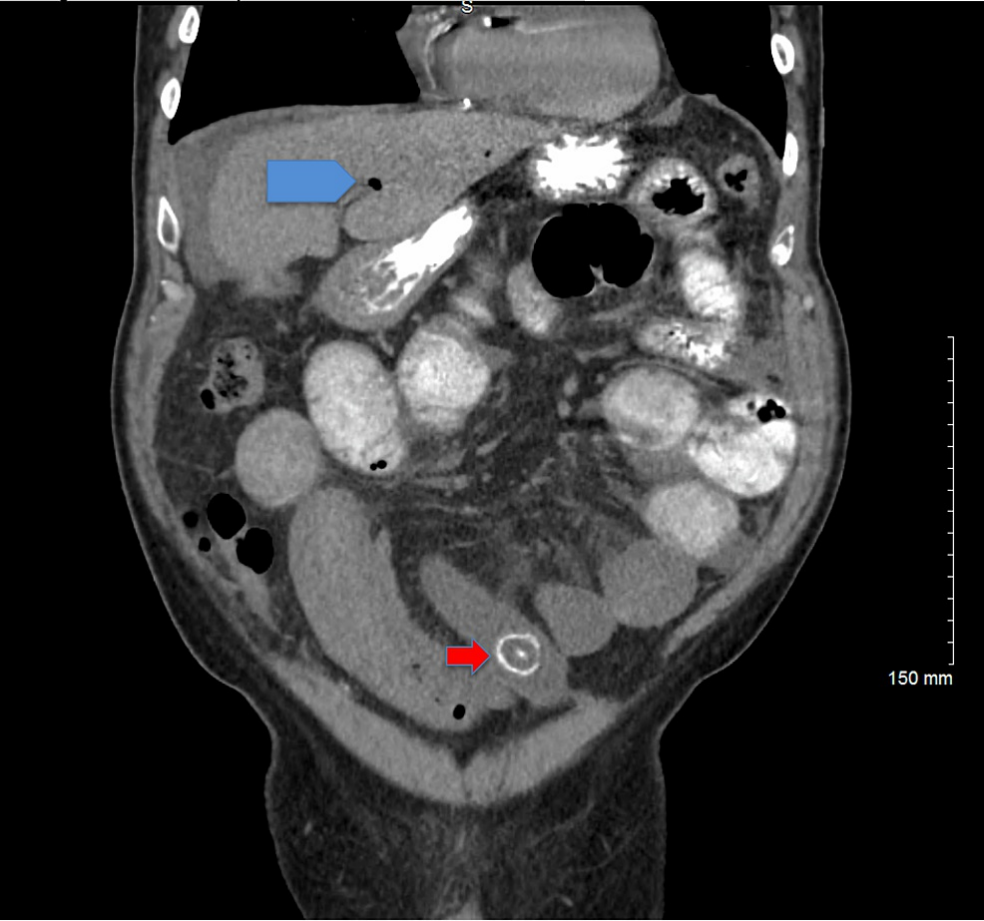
Coronal CT of abdomen and pelvis showing dilated small bowel loops, pneumobilia (blue pentagon), and impacted gallstone in the small bowel (red arrow).
